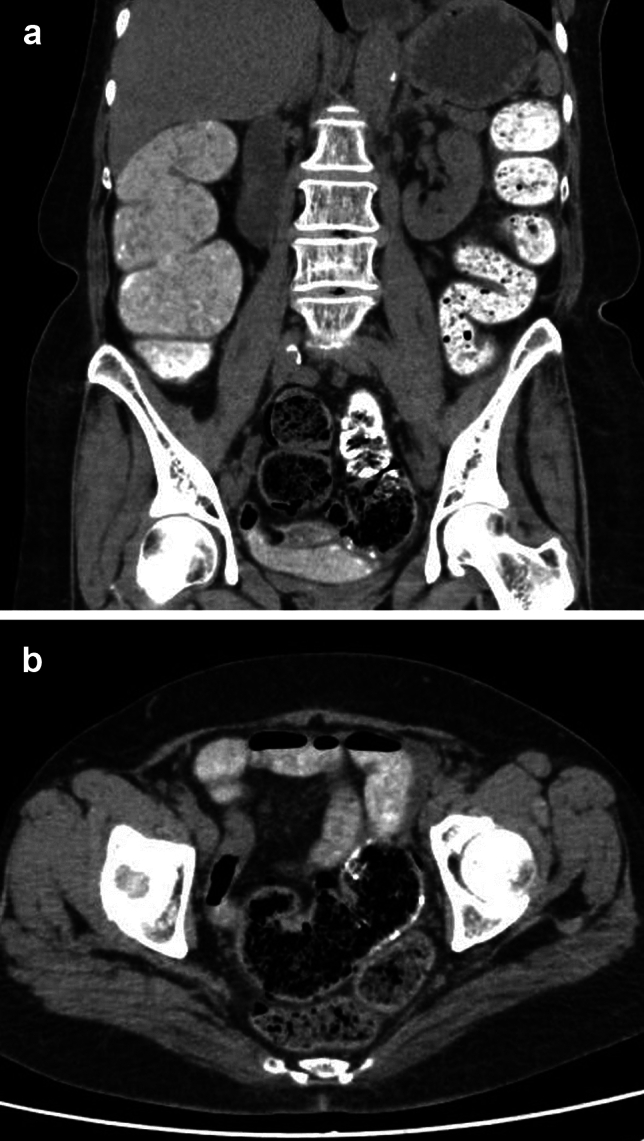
A 66-year-old female was scheduled for CT colonography to investigate the cause of her constipation. She took a bowel preparation agent containing barium starting the day before the examination, but no bowel movements occurred. On the day of the examination, she presented with fever, abdominal pain, and low blood pressure, entering a state of shock and requiring hospitalization. a, b Tagged stool was observed from the ascending colon through the sigmoid colon, while untagged stool was found in the rectum. The patient was diagnosed with sepsis secondary to obstructive colitis and died the following day
